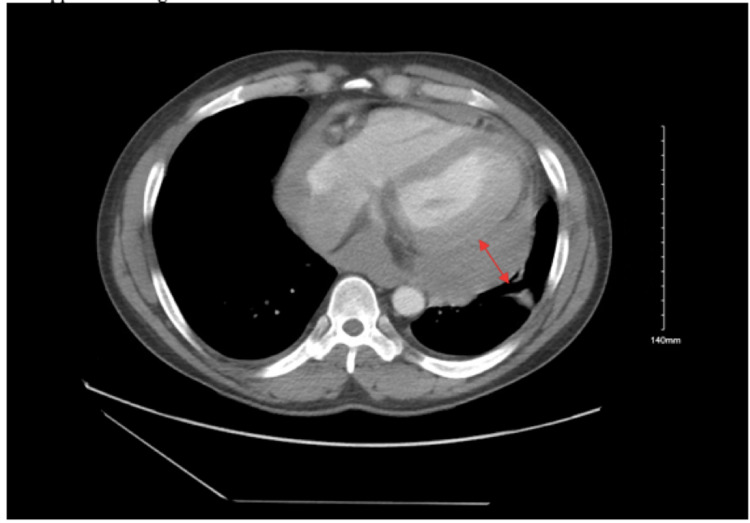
CT of the chest with contrast (axial view) showing posterolateral loculated pericardial effusion, which measures 36 mm.
